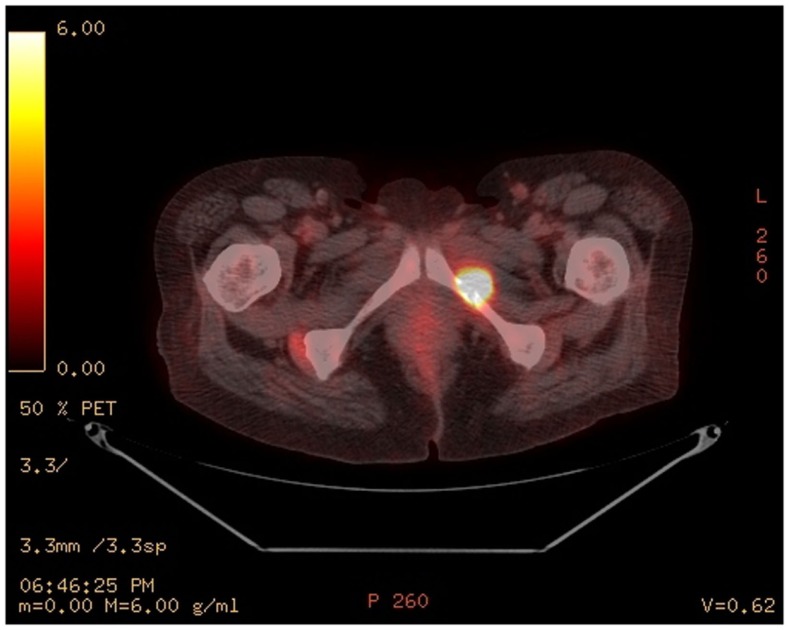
Positron emission tomography/computed tomography of the pelvis showed a hypermetabolic lytic lesion with a pathologic fracture involving the left inferior pubic ramus with maximum SUV 15.1 associated with a soft tissue mass 2.5 cm. × 1.8 cm.
